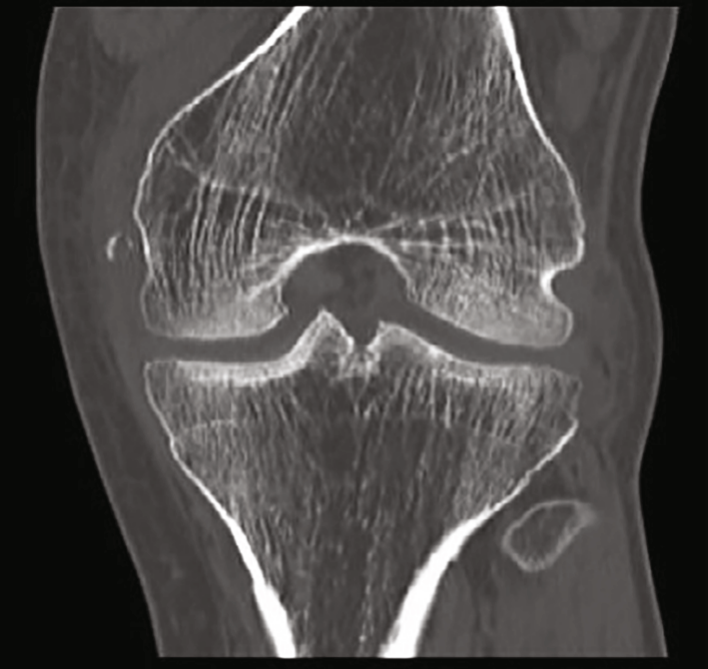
CT scan of Case 2. The coronal scan shows femoral Segond fracture at the proximal insertion of LCM.
